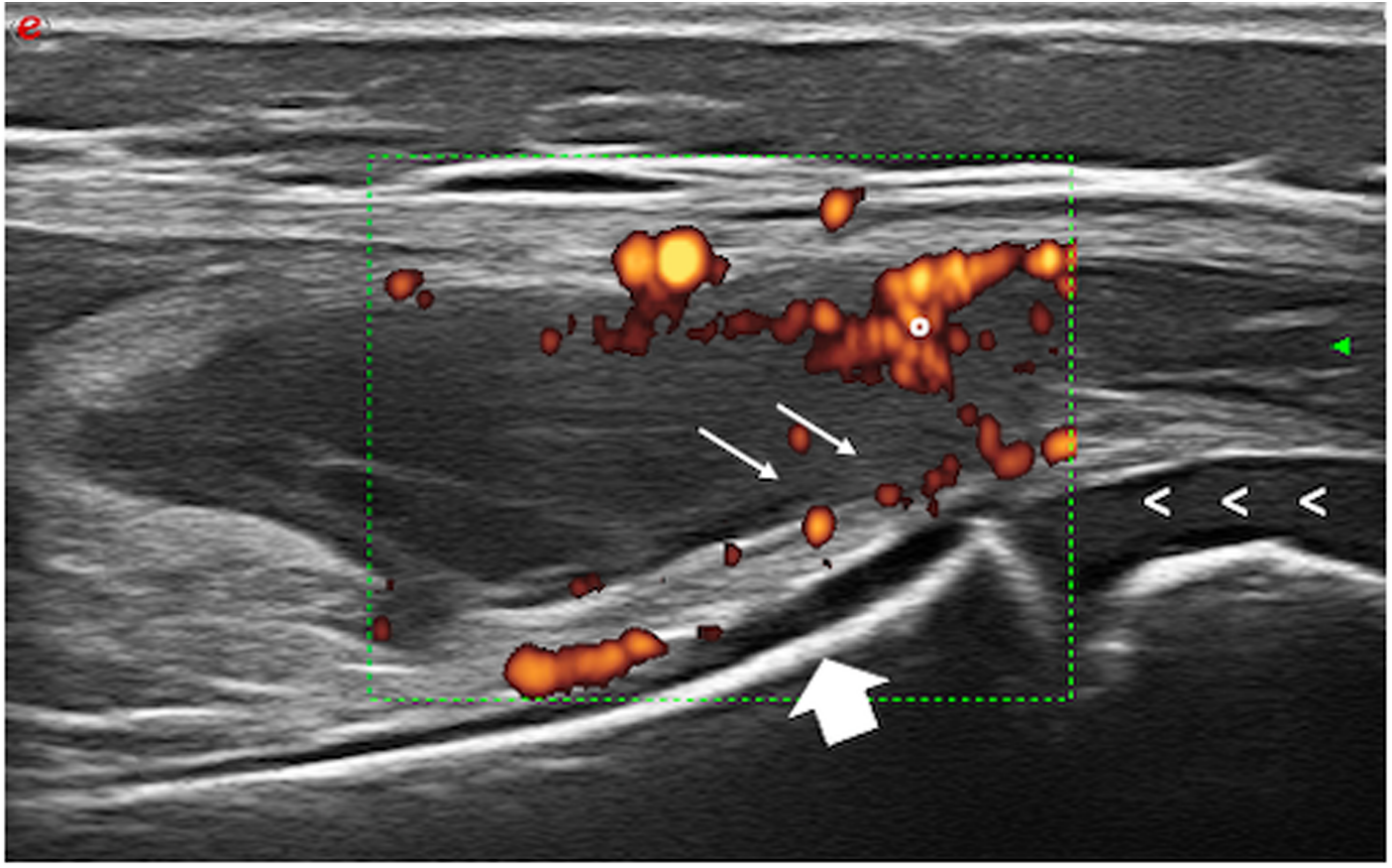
Knee ultrasound of case 2 showing active synovitis. °, Power Doppler signal of synovial tissue; arrows, synovial hypertrophy; <, growing cartilage; big arrow, bone.
